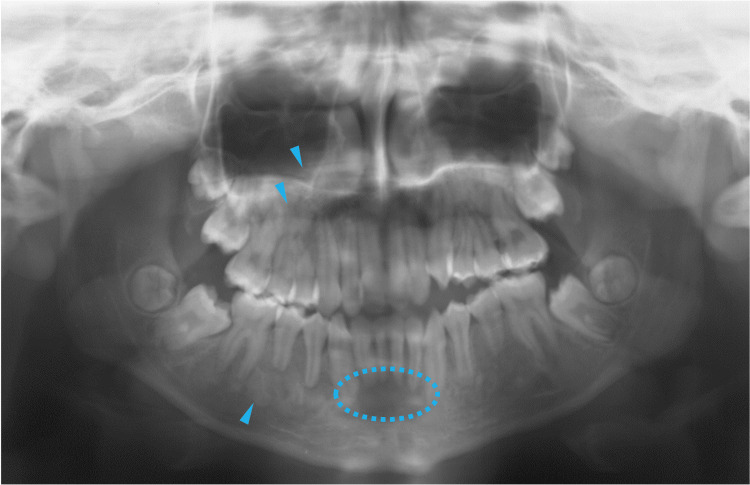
Maxillofacial radiograph at age 12 Blue arrowheads indicate the opacities in the maxillary sinus and mandibular premolar regions. The blue dotted circle indicates the opacity in the anterior mandible region
